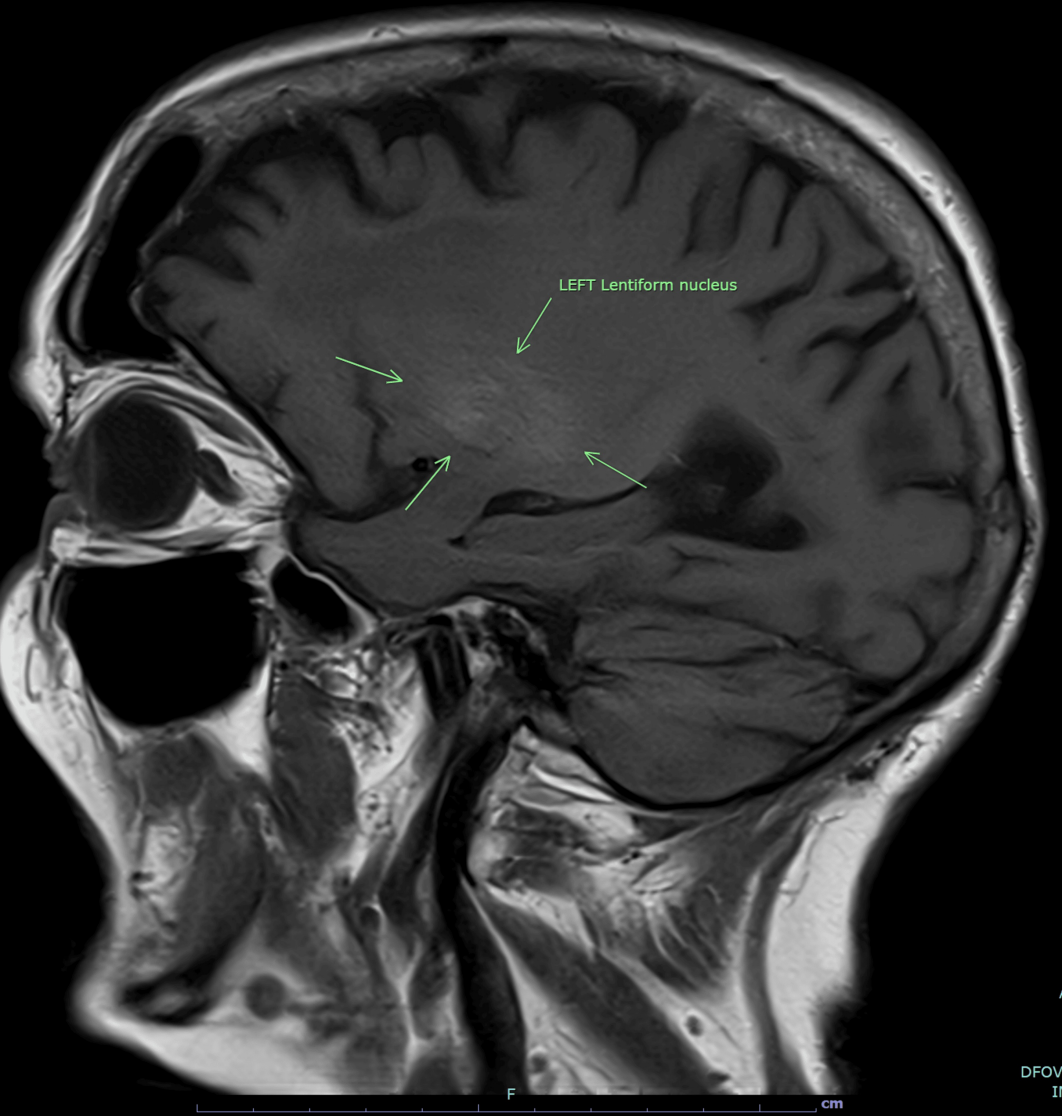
MRI brain T-1 parasagittal sequence image Slight hyperintensity noted in the area of the left lentiform nucleus, as pointed by the green arrows.
